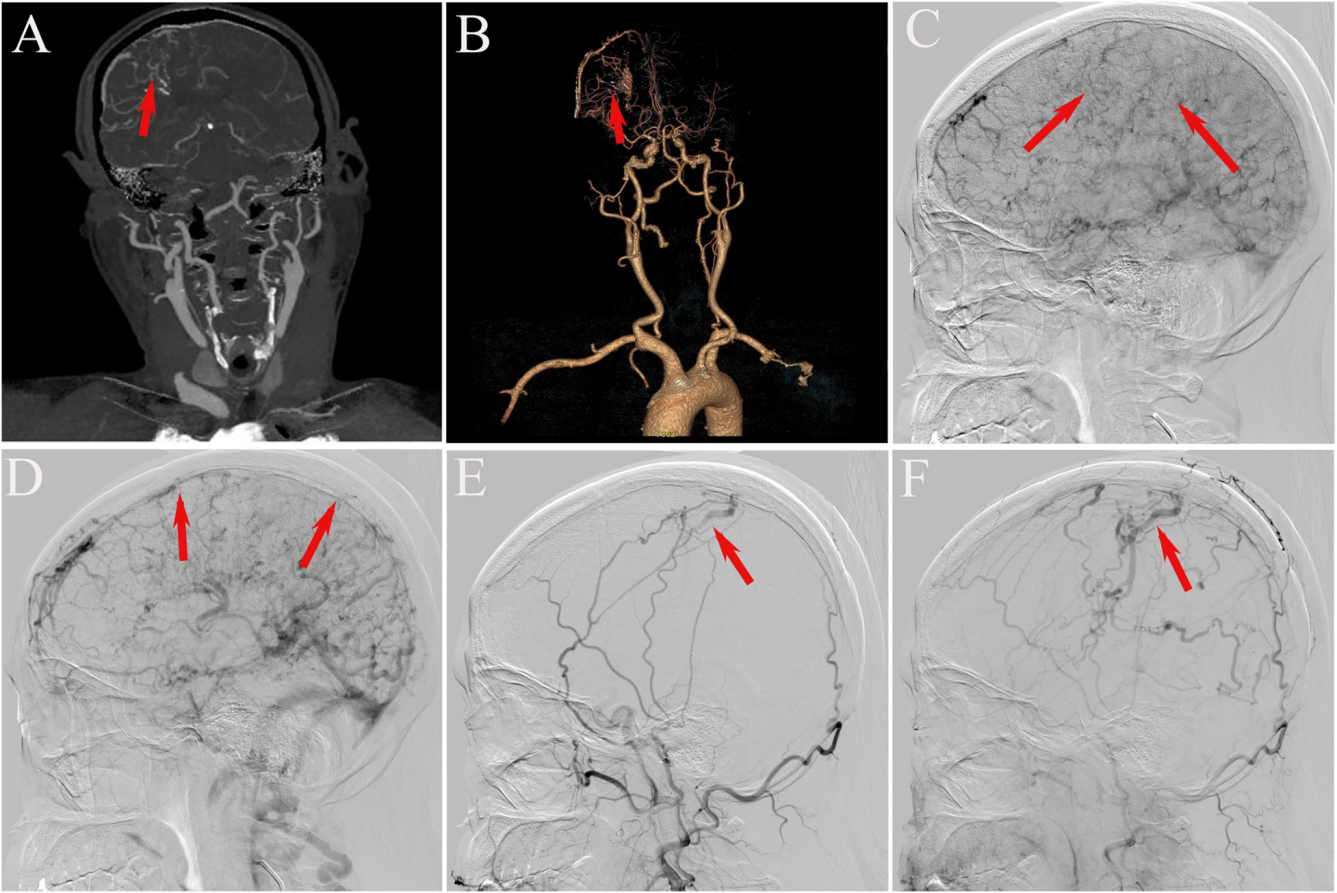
(A,B) head and neck vascular CTA revealed numerous slender and tortuous vascular structures within the bilateral brain parenchyma, with a predominance on the right side, as well as dilated vascular structures beneath the right cranial plate (red arrow). (C) Imaging of the right internal carotid artery revealed a dilated and disorganized right cerebral venous system during the venous phase (red arrow). (D) The absence of the superior sagittal sinus in the venous sinus phase indicated occlusion of this sinus (red arrow). (E,F) Bilateral external carotid arteriograms demonstrated abnormal dural vasculature draining through the cortical veins of the right parietal lobe, with an abnormally dilated draining vein in the cranium (red arrow).
